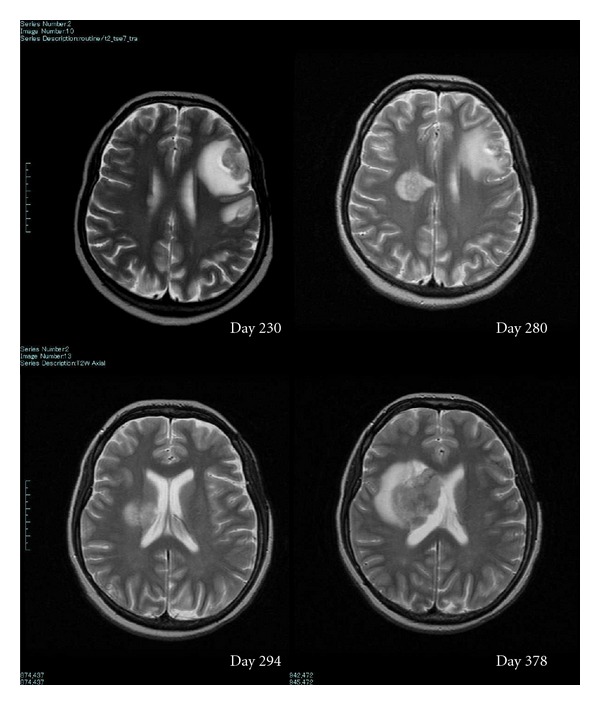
Brain MRI of the patient. Initial frontal tumor that was surrounded by T2 high edematous area disappeared completely on the 294th day, but right paraventricular tumor grew progressively.
